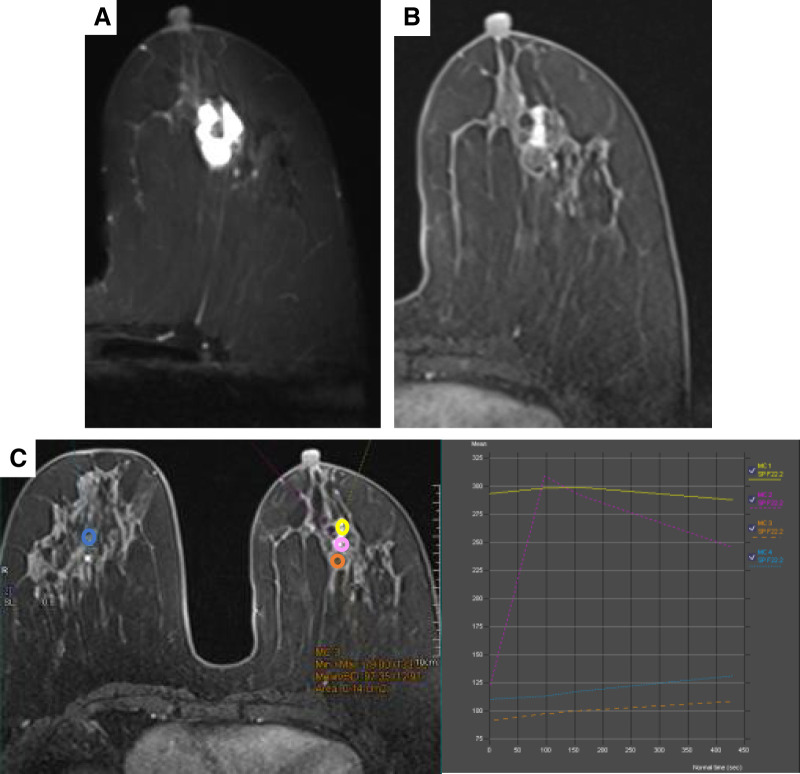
(A) In the T2-weighted MRI, areas other than the center exhibit high signal intensity. (B) On dynamic MRI, contrast enhancement is observed in the central part of the tumor. (C) The time–intensity curve of the enhanced central region (yellow, orange, and pink) exhibits different enhancement patterns, including rapid plateau and normal patterns.
